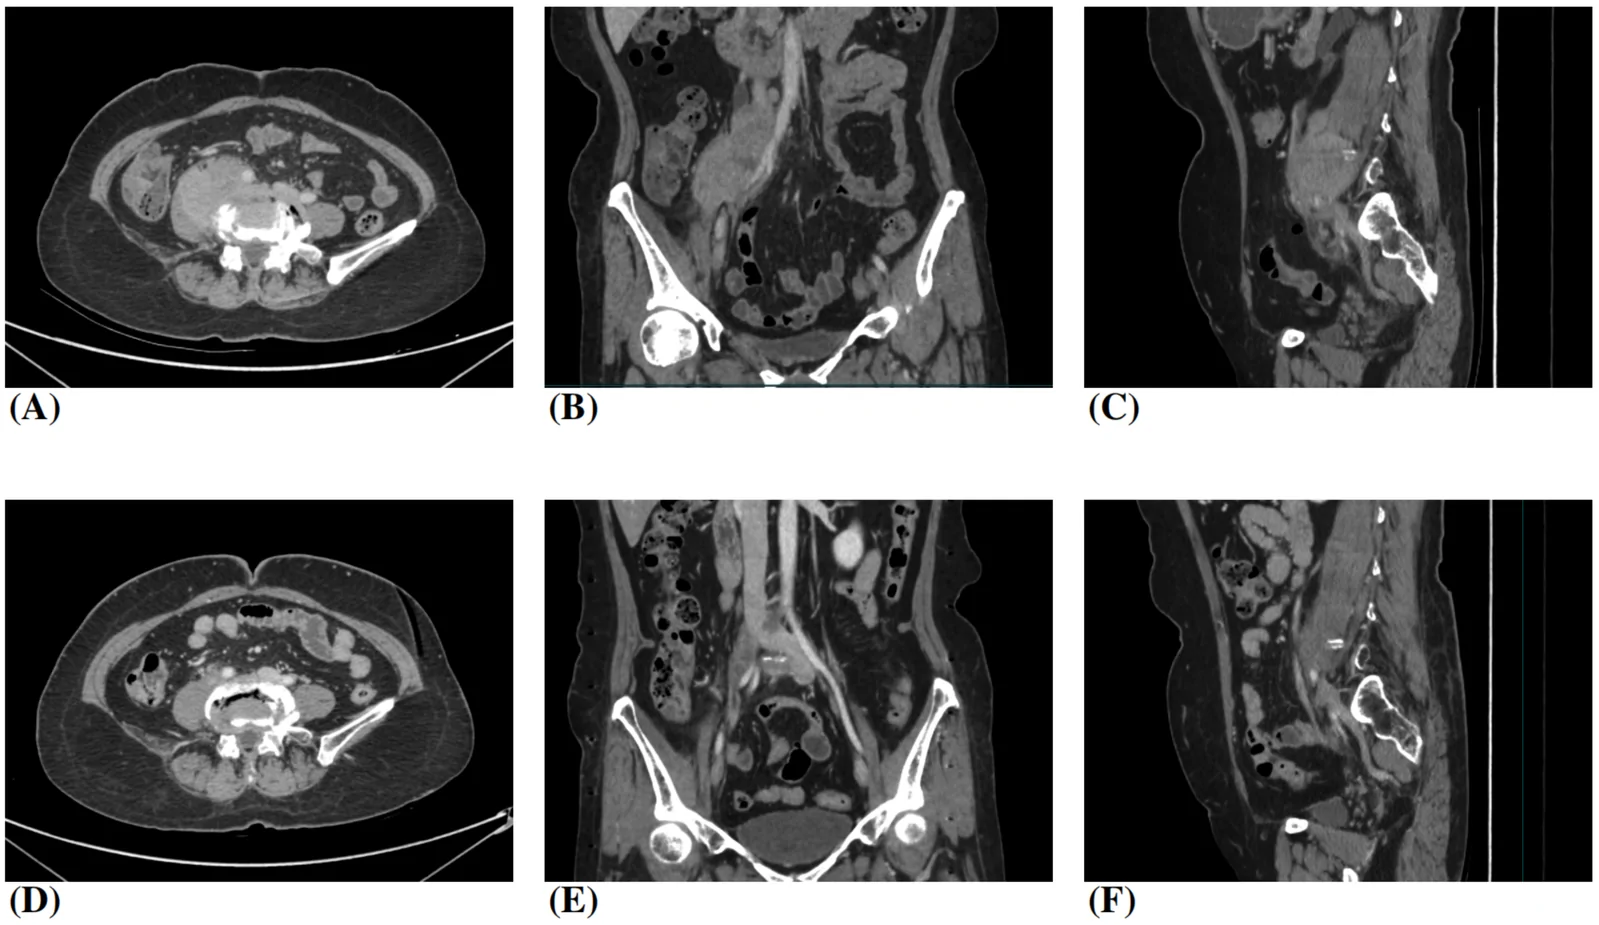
Pre- and post-radiotherapy CT comparison for retroperitoneal IMT. (A) Pre-radiotherapy axial CT slice showing the retroperitoneal IMT lesion. (B) Pre-radiotherapy coronal CT slice showing the retroperitoneal IMT lesion. (C) Pre-radiotherapy sagittal CT slice showing the retroperitoneal IMT lesion. (D) Post-radiotherapy axial CT slice showing the retroperitoneal IMT lesion. (E) Post-radiotherapy coronal CT slice showing the retroperitoneal IMT lesion. (F) Post-radiotherapy sagittal CT slice showing the retroperitoneal IMT lesion.
